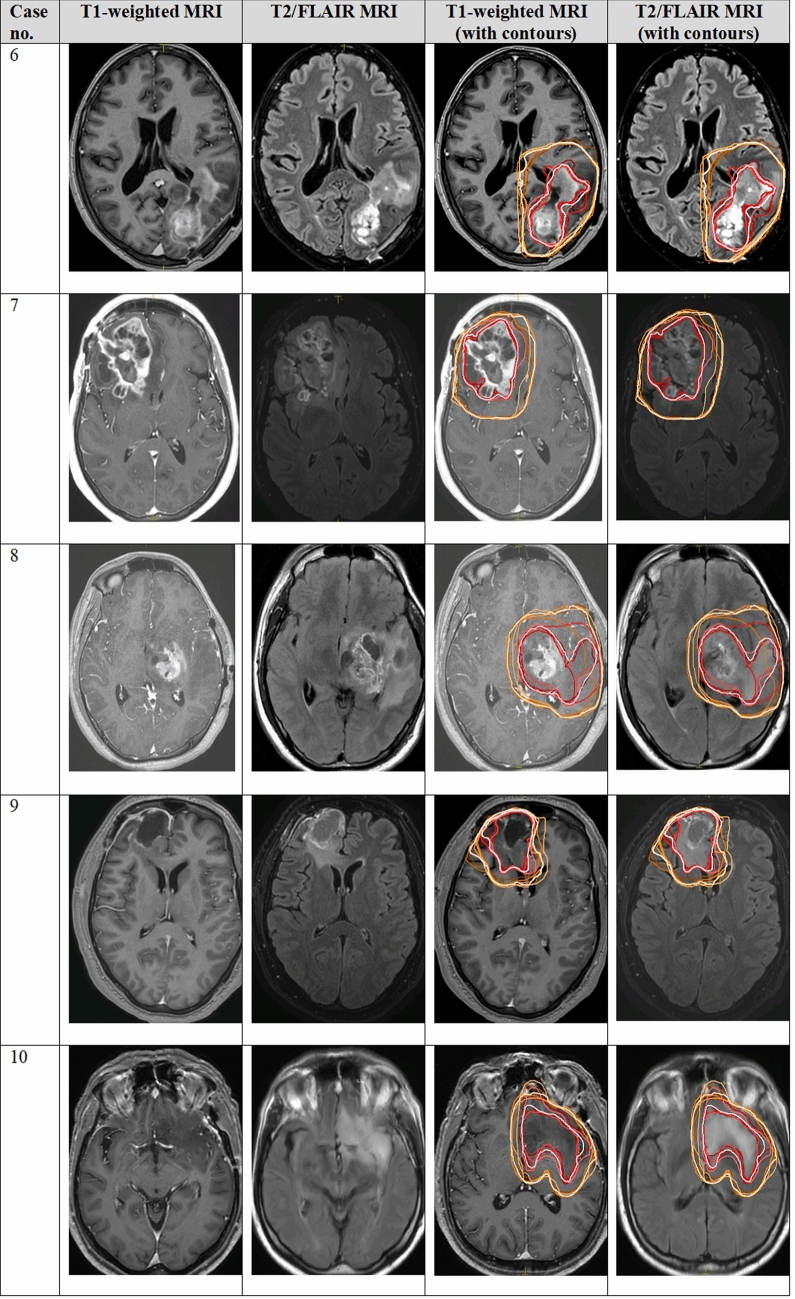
Individual physician and consensus target volume contours on selected axial slice for the GTV and CTV in low and high grade gliomas, cases 6–10. Consensus contours are shown in thick white and individual contours in shades of other colors. *MRI* magnetic resonance imaging, *GTV* gross tumor volume, *CTV* clinical target volume
